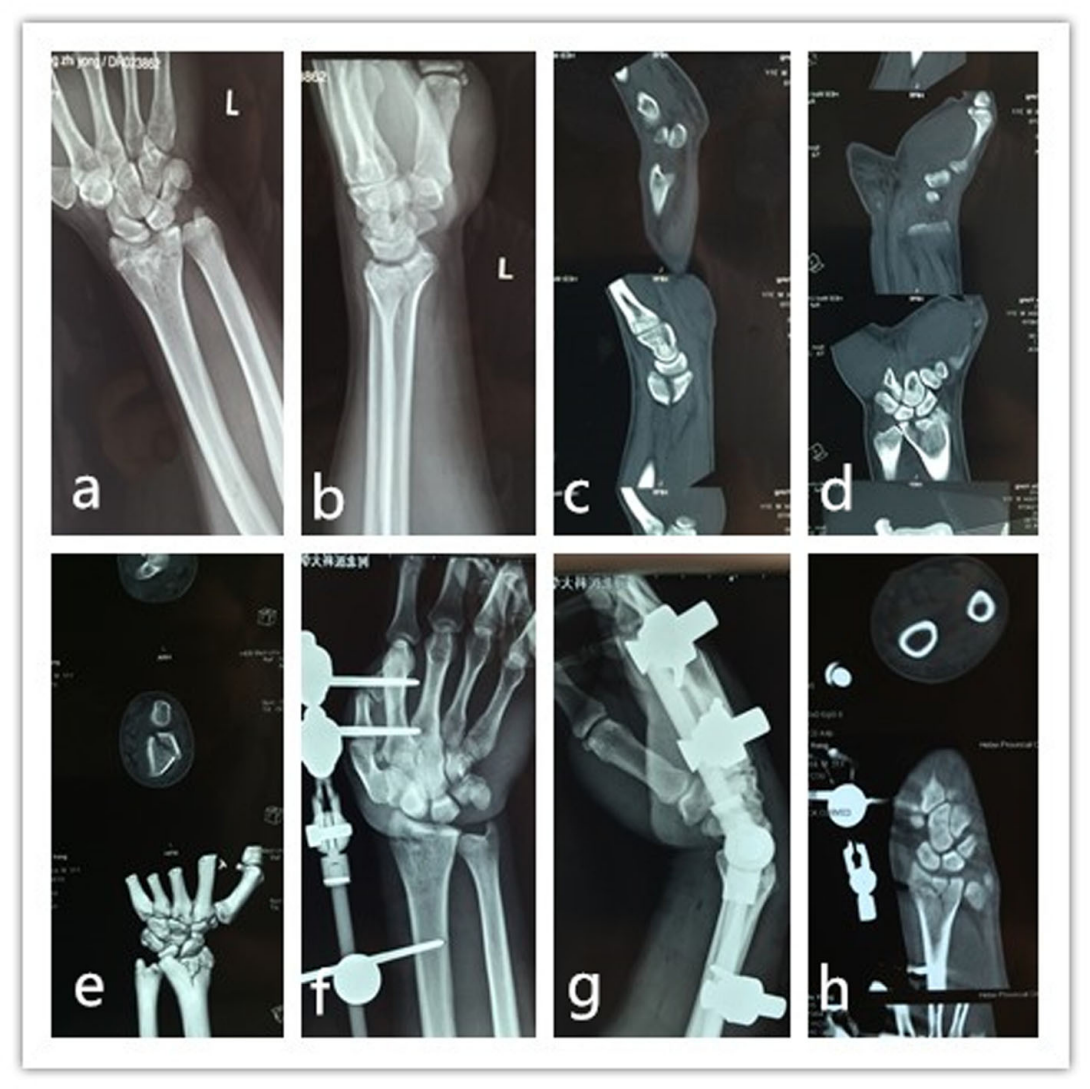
A male patient, 44 years old, sustained intra-articular fracture of right distal radius. In the outpatient, he was diagnosed as die-punch fracture based on radiographs (anteroposterior, a and lateral, b) and CT scanning (c–f). Finally, he was treated by VLP (g, h) and the CT scanning showed favorable reduction and fixation
